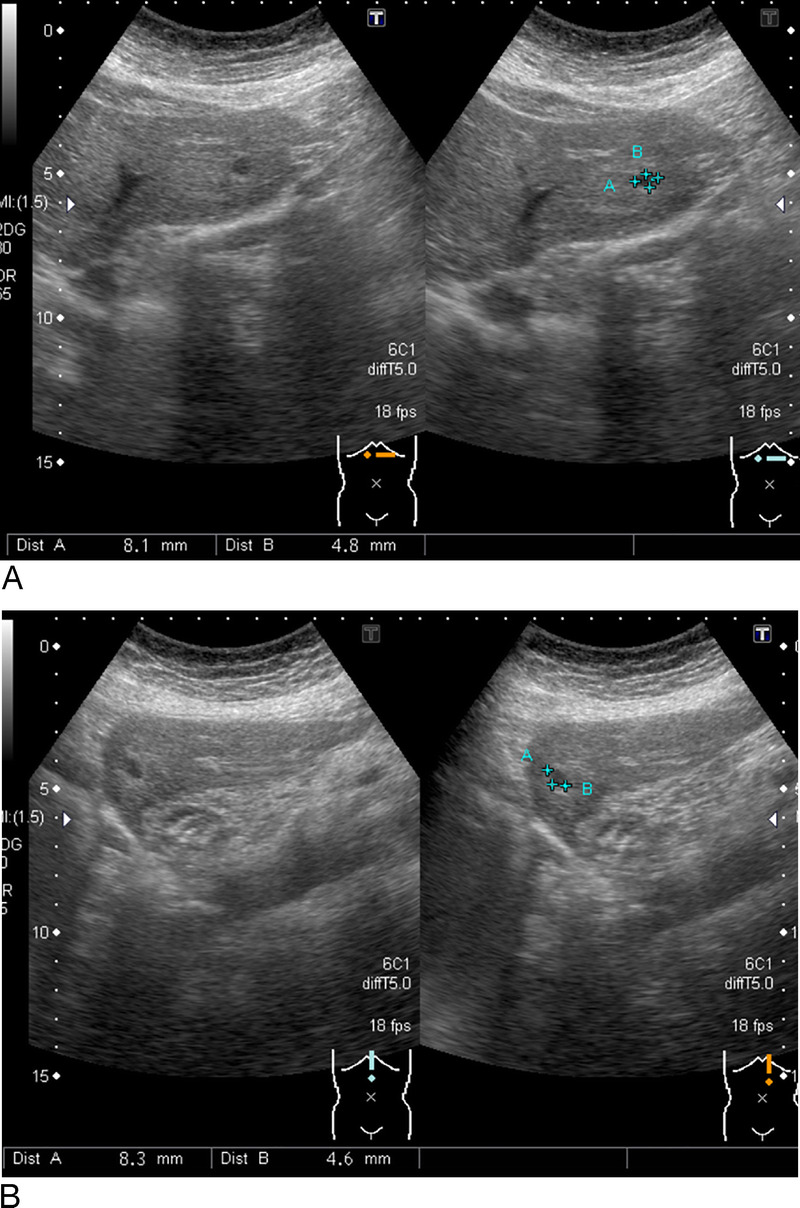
Ultrasound shows an 8-mm hypoechoic mass in the left lateral section of the liver on initial evaluation (A, B).
